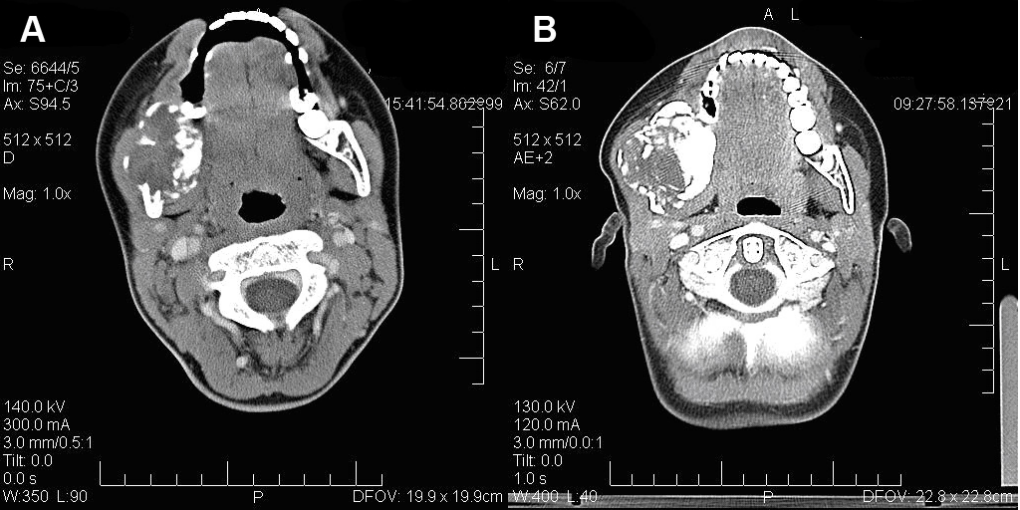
Preoperative computed tomography scan in comparison to the scan at first admission, revealing the tumour progression. A: CT before treatment: In the right mandibular angle a heterogenous mass of 3.0 × 3.6 cm is demonstrated, showing a rather sharp margin with cortical destruction. B: CT three months later: The computed tomography scan three months later reveals progression of the tumour mass (4.5 cm), with a further ballooning of the cortical structure and an increase of hard tissue, some osteolytic lesions and a central soft tissue component.
